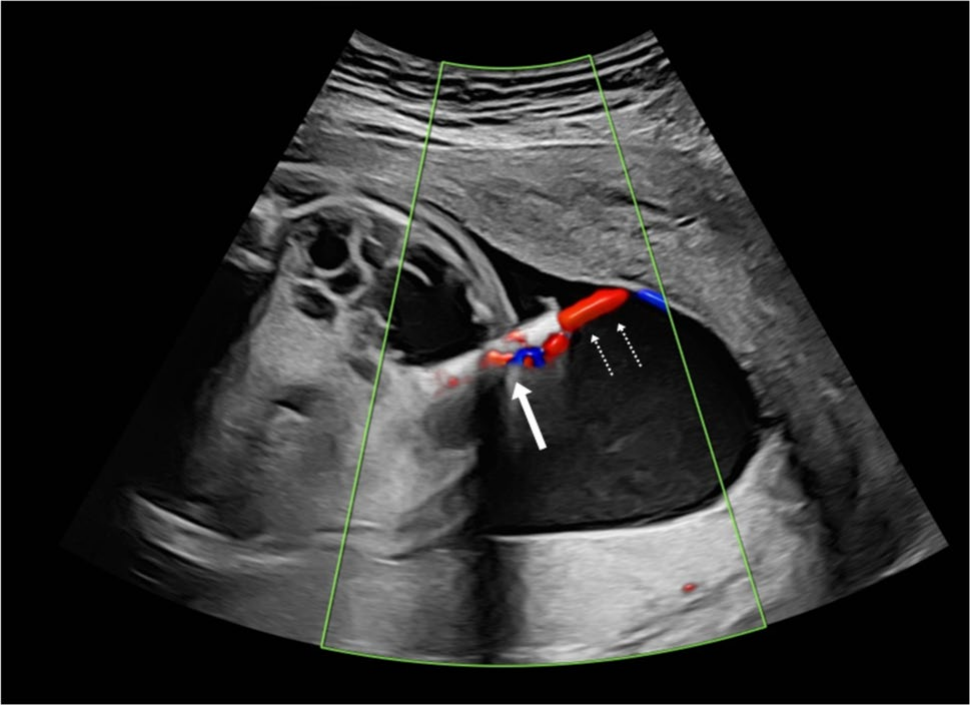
Somatex^®^ intrauterine shunt (arrow) seconds after placement in the largest cyst of a right sided CPAM at 26 weeks. The evacuation of fluid (dotted arrow) is demonstrated by color Doppler
